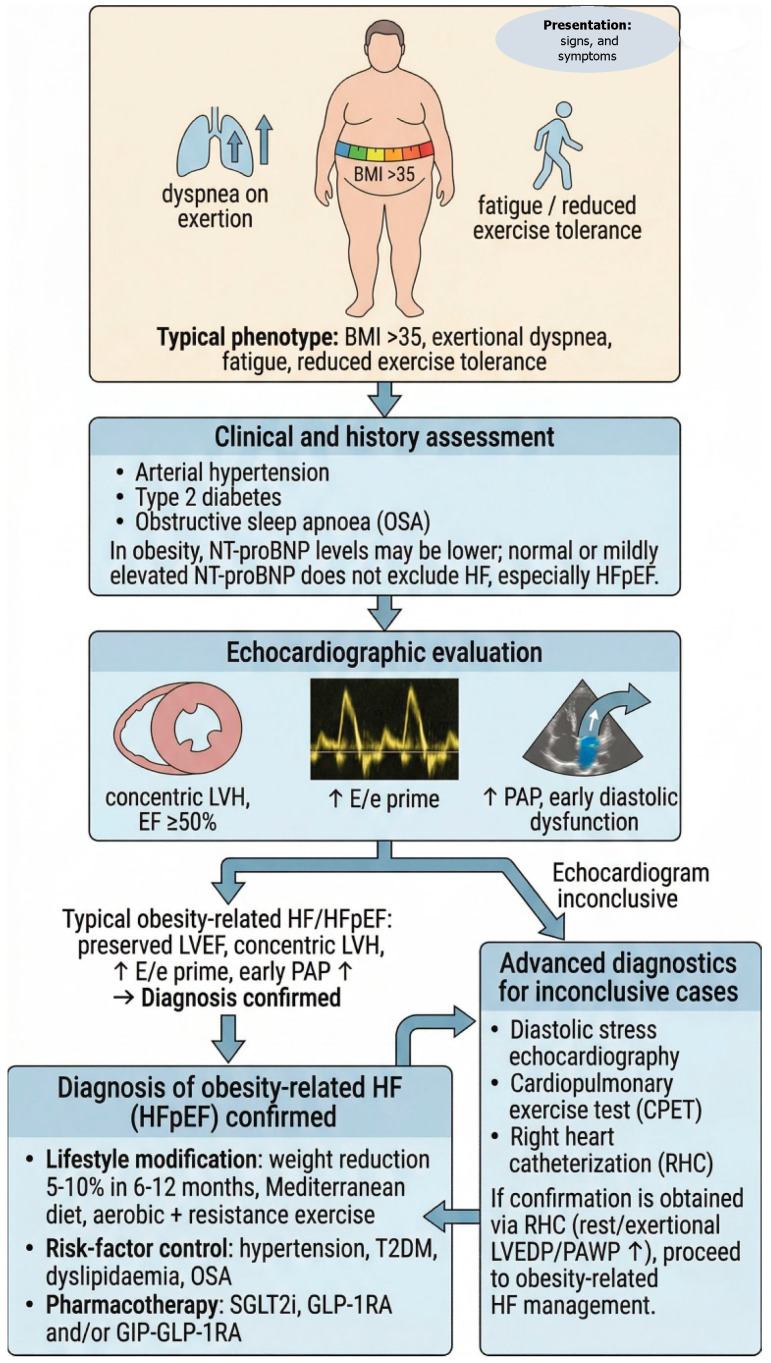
This presents a stepwise diagnostic and management algorithm for obesity-associated heart failure, a phenotype predominantly characterized by HFpEF. It emphasizes careful clinical assessment, cautious interpretation of natriuretic peptides, comprehensive echocardiographic evaluation, and functional testing when needed to confirm elevated filling pressures. Management integrates guideline-directed heart failure therapy with aggressive comorbidity optimization and structured weight reduction, recognizing obesity as a central driver of the syndrome [30,31,32,33,34]. List of Abbreviations: BMI, Body Mass Index; HF, Heart Failure; HFpEF, Heart Failure with Preserved Ejection Fraction; LVEF, Left Ventricular Ejection Fraction; LVH, Left Ventricle/Left Ventricular Hypertropia; E/e, ratio of Early mitral inflow velocity to early diastolic mitral annular velocity; PAP, Pulmonary Artery Pressure; NT-proBNP, N-Terminal pro-B-type Natriuretic Peptide; T2DM, Type 2 Diabetes Mellitus; OSA, Obstructive Sleep Apnea; SGLT2i, Sodium–Glucose Cotransporter type 2 inhibitors; GLP-1RA, glucagon-like peptide-1 receptor agonists; GIP, glucose-dependent insulinotropic polypeptide; CPET, CardioPulmonary Exercise Test; RHC, Right Heart Catheterization; LVEDP, Left Ventricular End-Diastolic Pressure; PAWP, Pulmonary Artery Wedge Pressure. ↑, increase.
